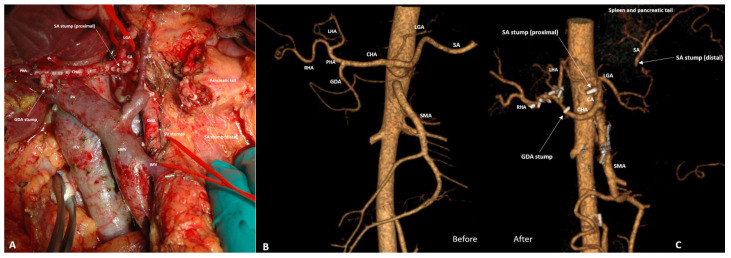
Pylorus-preserving pancreaticoduodenectomy with splenic vessels resection for BR PHDAC in a 54-year-old lady. (A) An operating field after completion of the resection stage. CTA before (B) and after (C) surgery, showing the sources of blood supply to the pancreatic tail and spleen from the branches of the LGA after splenic vessels resection. The diameter of LGA increased by two times, increasing the VBF through the LGA by 16 times.
